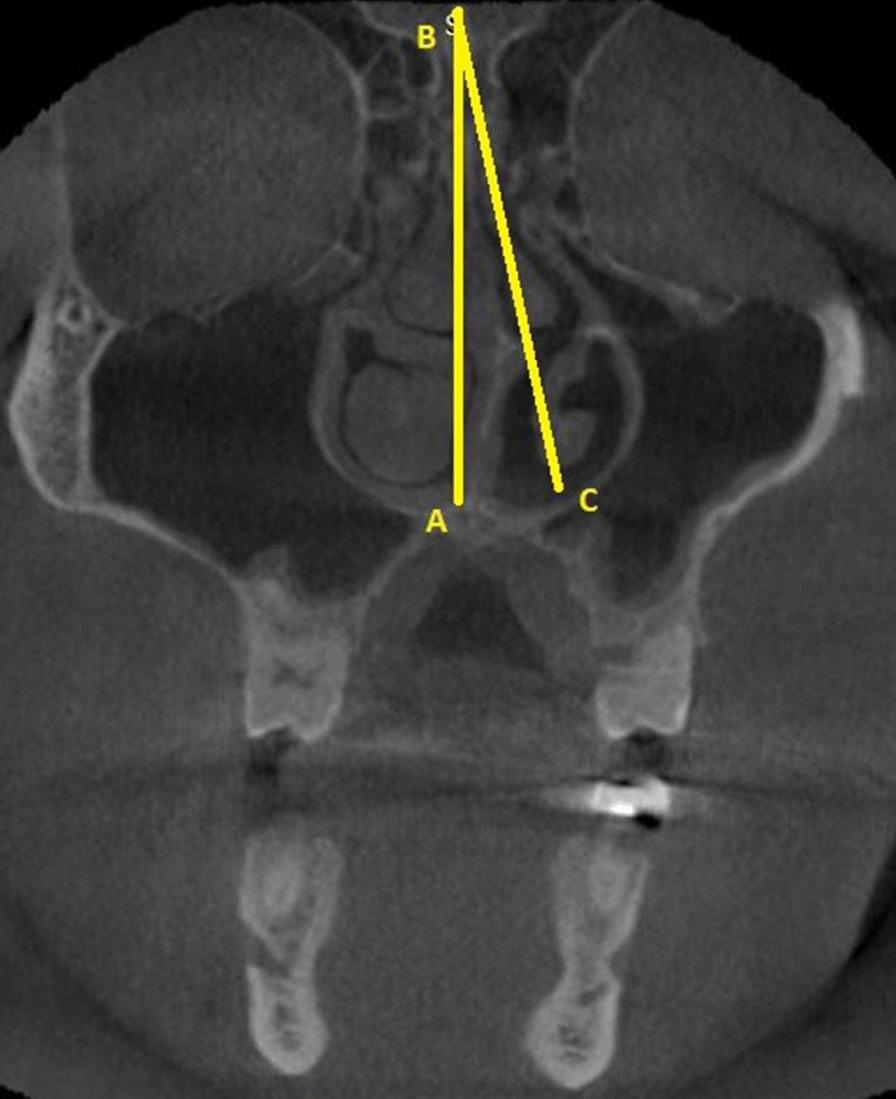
Coronal CBCT section showing method used for calculating septal deviation angle SDA
